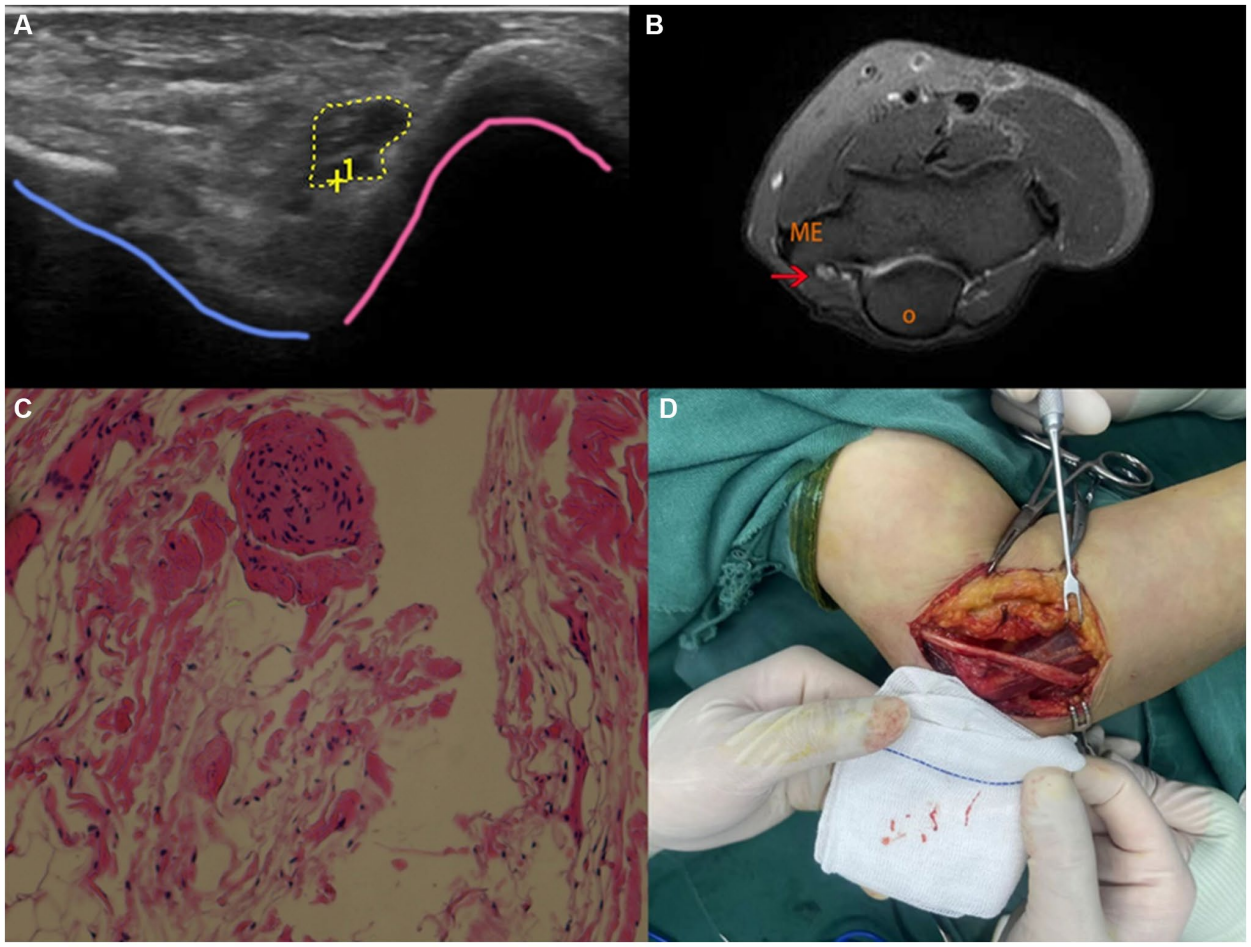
US, MRI, pathological examination and intraoperative images of a 54-year-old female patient suffering from numbness and tenderness on the ulnar side of left hand. (A) US imaging on the left elbow showed hypoechoic thickening of the nerve fascicles at the cubital tunnel; (B) MRI showed significant thickening of the left ulnar nerve at the cubital tunnel with increased T2-weighted signal intensity. Red arrow marks the ulnar nerve swelling. O (olecranon), ME (medial epicondyle). (C) Pathological examination indicated small piece of fibrofatty tissue; (D) intraoperative image showed adhesion and edema of the ulnar nerve and surrounding tissue.
